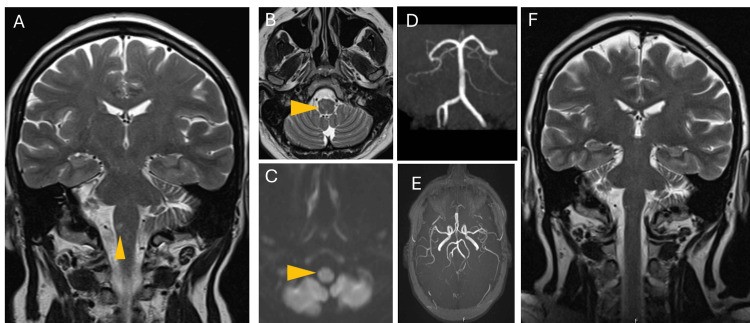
Brain MRI. T2-weighted in coronal (A) and axial (B) slices revealing a hyperintense lesion, right laterobulbar, and slightly hyperintense on DWI sequence (C). MRA without signs suggestive of dissection or vasculitis (D, E). Subsequent coronal slice showing a shrunken bulbar lesion on T2 weighting (F).
